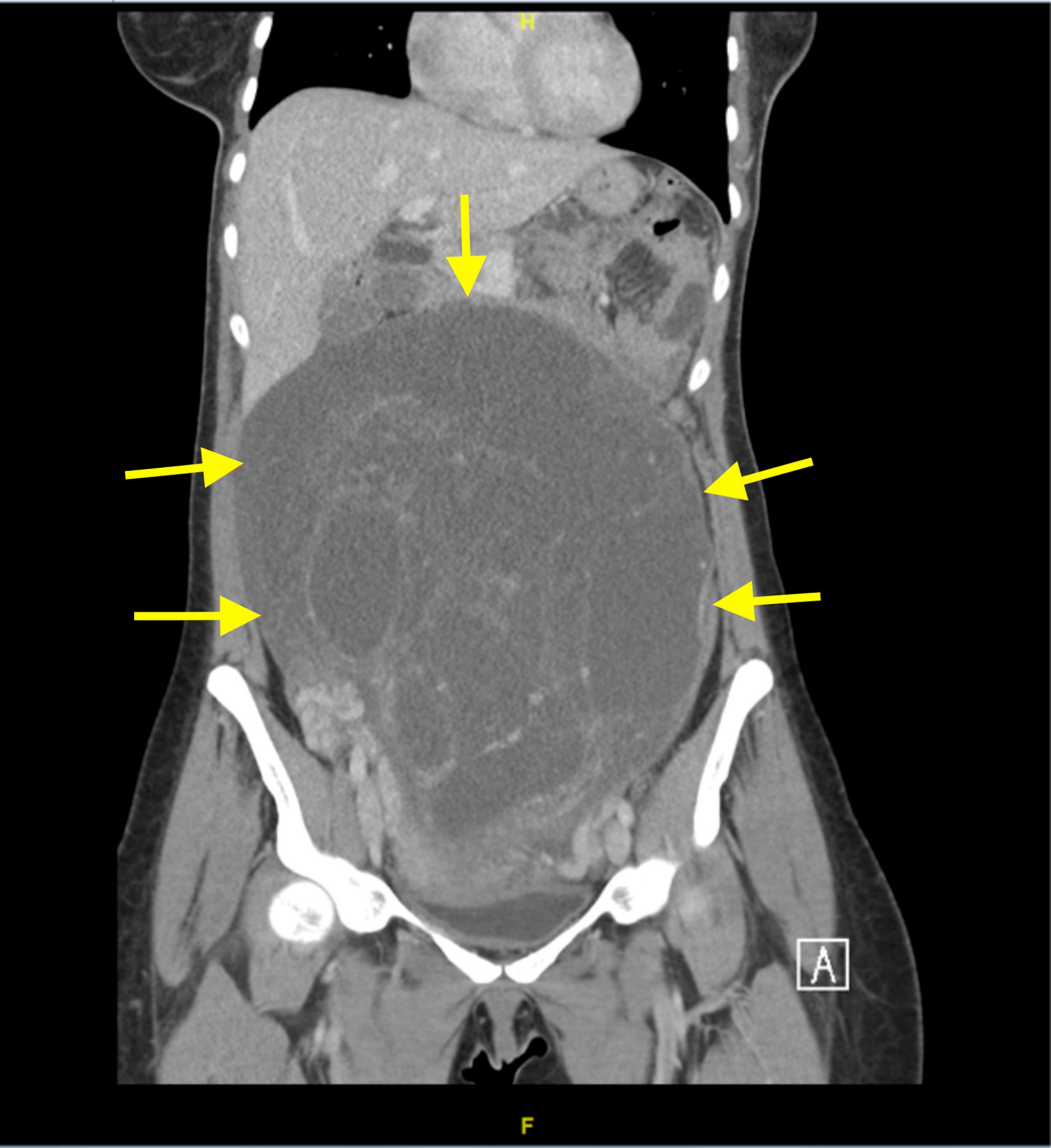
CT coronal view of the abdomen and pelvis Showing the large multiloculated abdominopelvic lesion.
